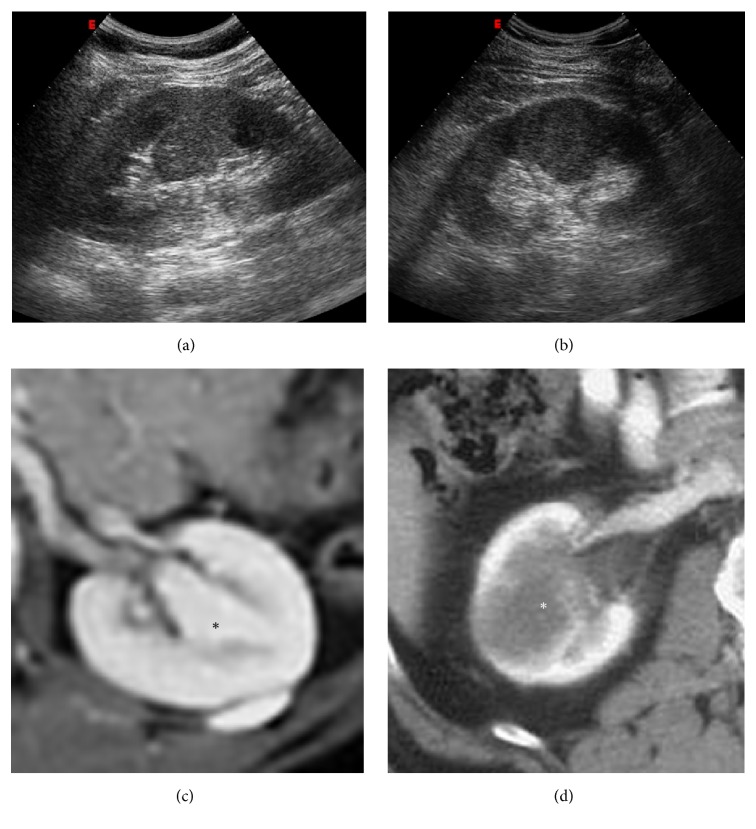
Pseudotumor versus true solid renal lesion. Gray scale US (a, b) and contrast-enhanced CT (c, d). Renal incidentalomas in two different patients with similar echogenicity pattern (a, b). Contrast-enhanced CT reveals a hypertrophied column of Bertin in the first case (black asterisk in (c)) and a solid hypovascular mass in the second case (white asterisk in (d)).
